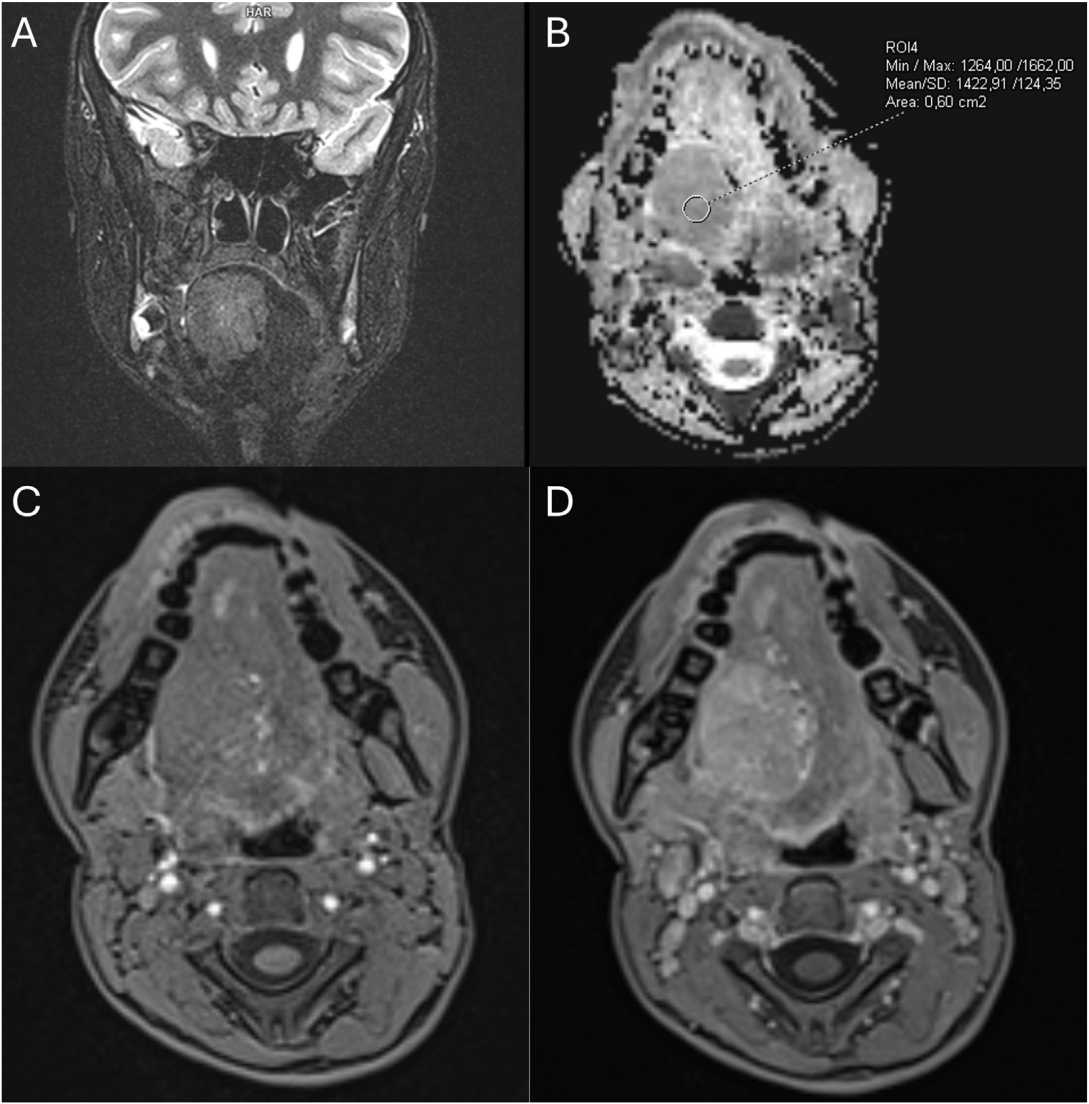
The first MRI examination in December 2021. (A) Coronal T2-weighted MRI image with fat saturation showing a pathologic mass in the tongue. (B) MRI-apparent diffusion coefficient (ADC) map highlighting the tongue mass. (C,D) Pre- and post-contrast T1-weighted gradient-echo MRI images demonstrating vivid enhancement of the tongue mass and dilated surrounding vessels.
